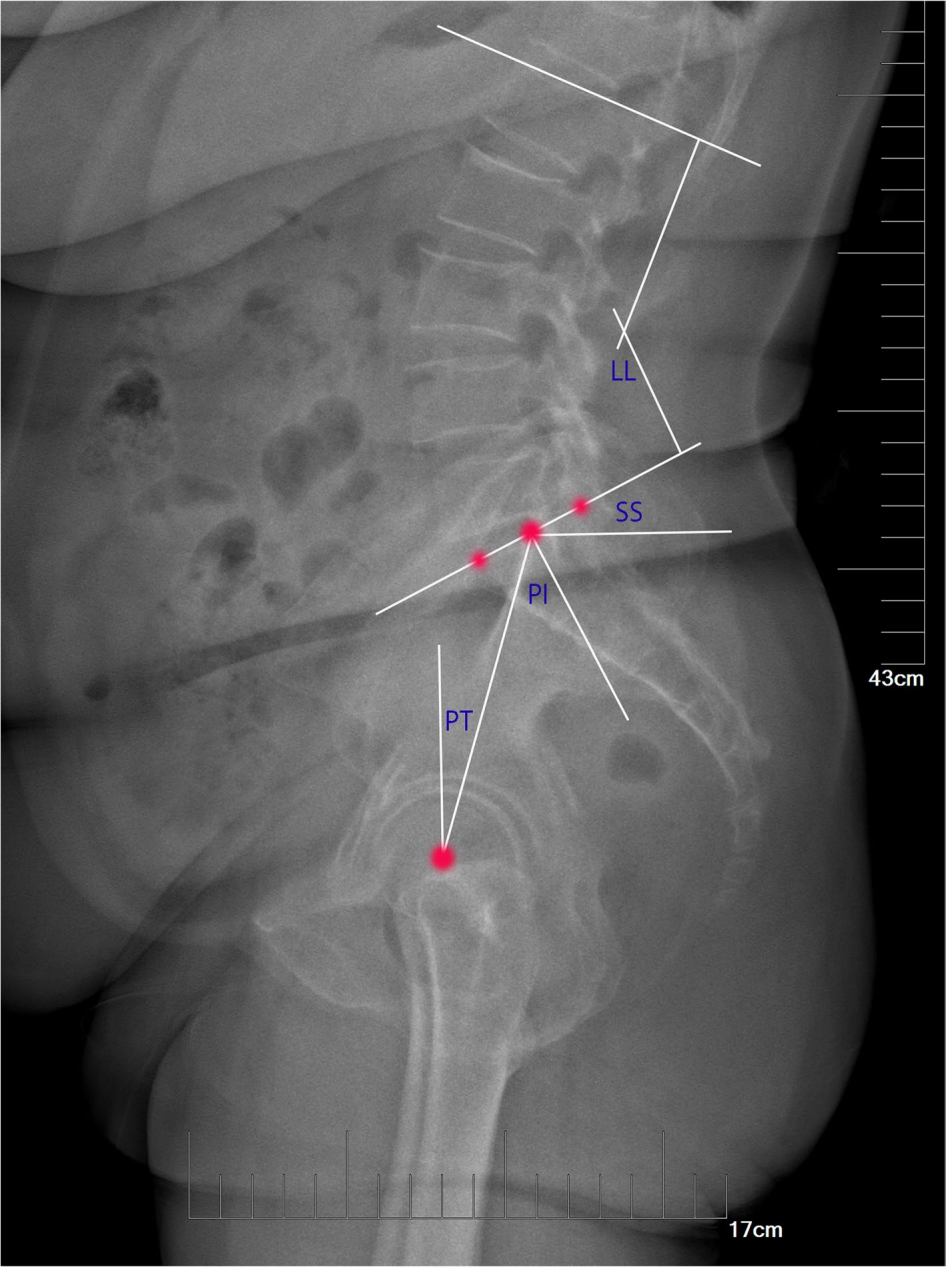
Plain lateral radiographs for measuring spino-pelvic sagittal plane parameters. LL: Lumbar lordosis; SS: Sacral slope; PI: Pelvic incidence; PT: Pelvic tilt
